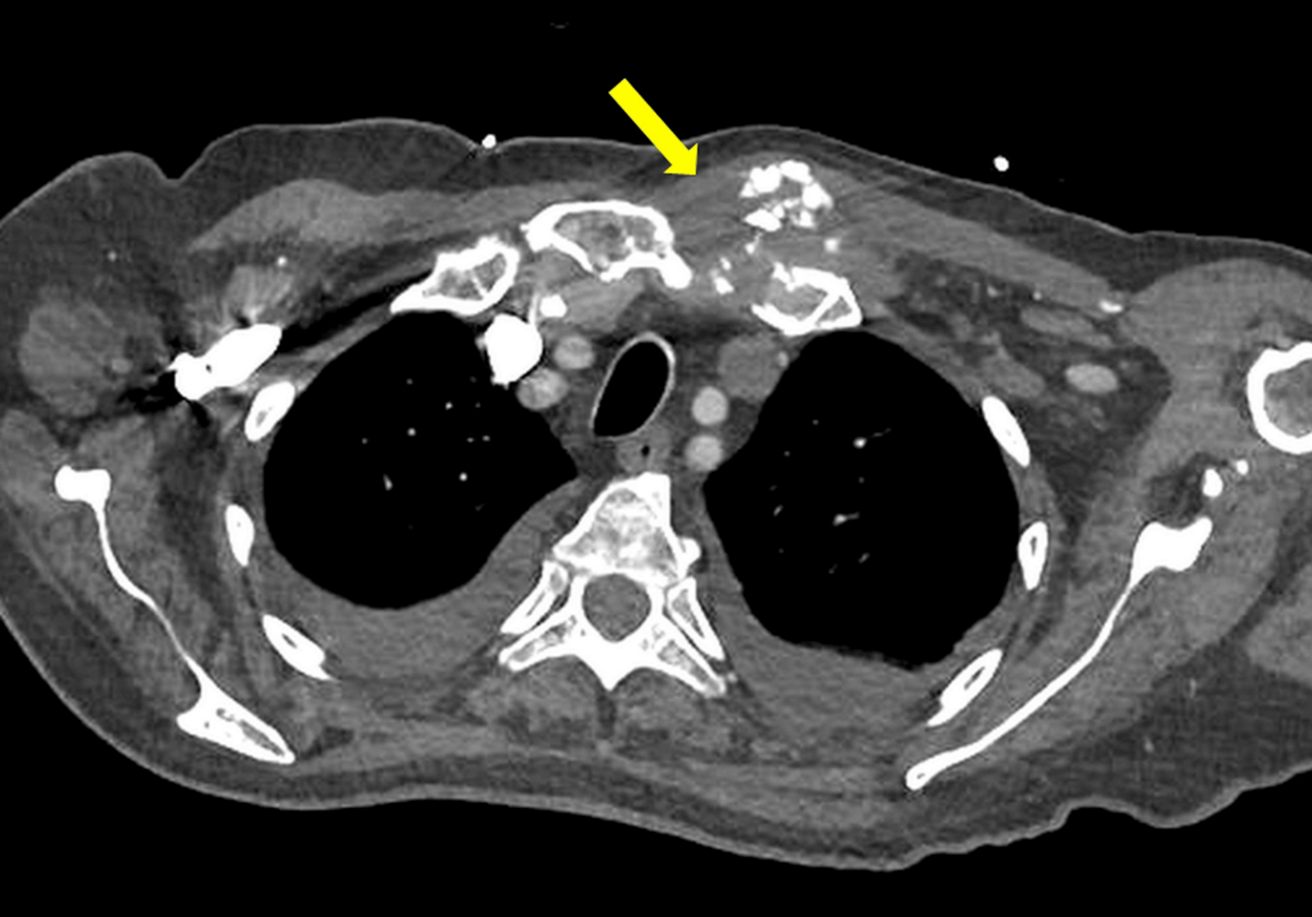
CT performed after treatment demonstrating the persistent bony destruction of the sternoclavicular joint (yellow arrow), which is expected following osteomyelitis, with no residual soft tissue collection
